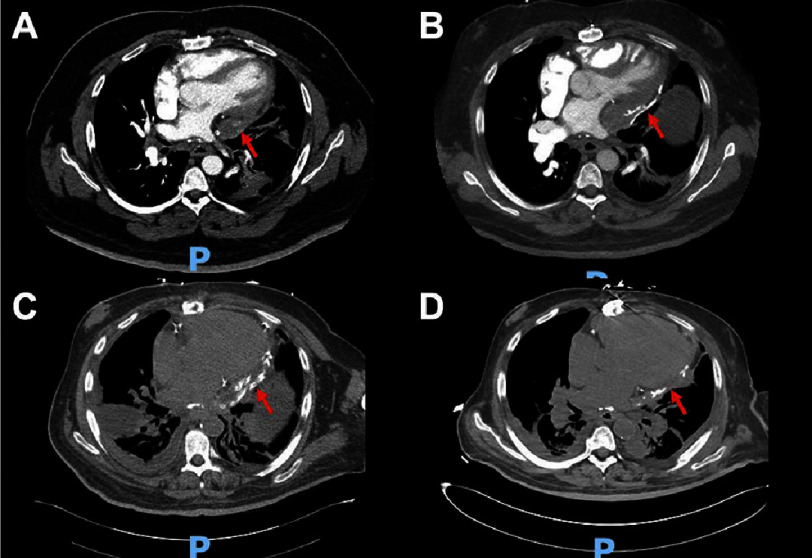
Computed tomography imaging of patient with saphenous vein graft aneurysm from time of diagnosis (A), after symptom onset (B), after first pericardiectomy (C), and after second pericardiectomy (D). The location of the saphenous vein graft aneurysm noted with red arrows. Letters are also noted in Figure 1 timeline.
